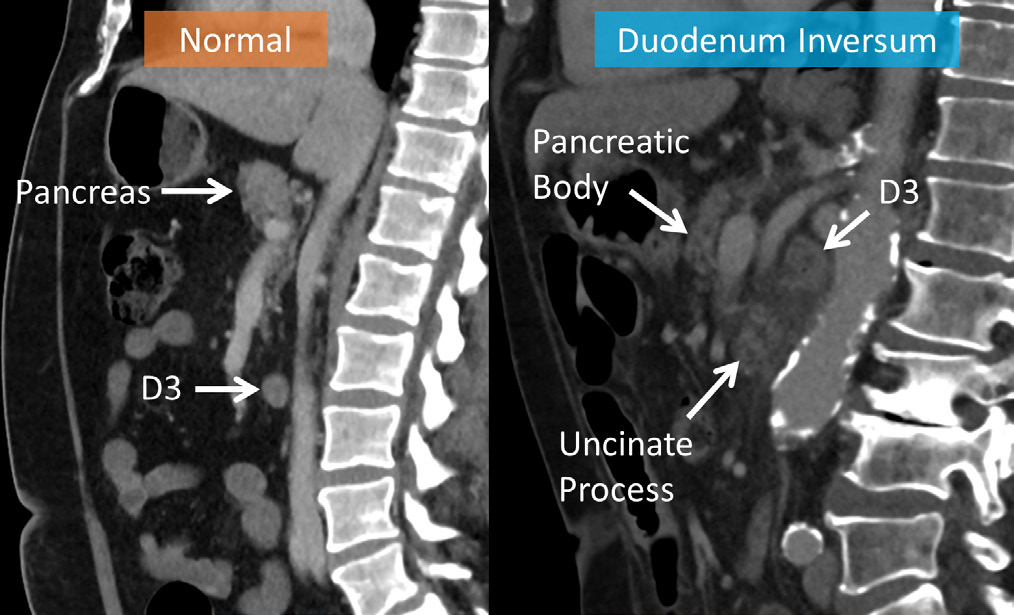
CT sagittal image demonstrating high positioning of the D3 segment of the duodenum in duodenum inversum compared to normal. Note the changes of duodenitis with abnormal wall thickening and surrounding fat stranding in the patient with duodenum inversum.
